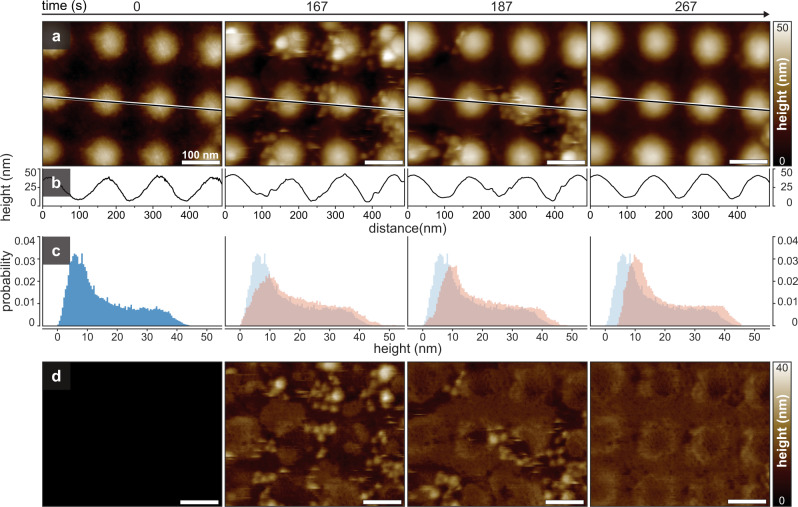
Time-lapse of SLB formation on undulated (concave-convex) nanopatterned HS-AFM supports. a HS-AFM frames (Supplementary Movie 1) of the bilayer formation process: *t* = 0 s: Non-flat sample surface before addition of lipid. *t* = 167 s: SUVs deposit on the surface. *t* = 187 s: Vesicles start to spread and fuse to form a SLB. *t* = 267 s: The entire surface is membrane covered. b Line profiles along the dashed lines in a. c Height distribution histograms of the images shown in a: Height values shifted over time by the thickness of a bilayer (~4 nm; compare blue and orange height distributions at *t* = 0 s and *t* = 267 s, respectively. d Nanopattern-subtracted images of the images shown in a. Source data are provided as a Source Data file.
